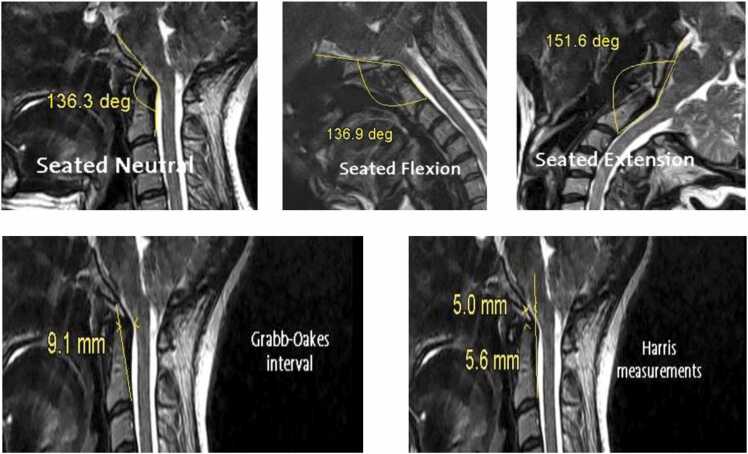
Upright MRI showing clivo-vertebral angle measurement ∼136° neutral; increased in extension, consistent with hypermobility-associated instability.
